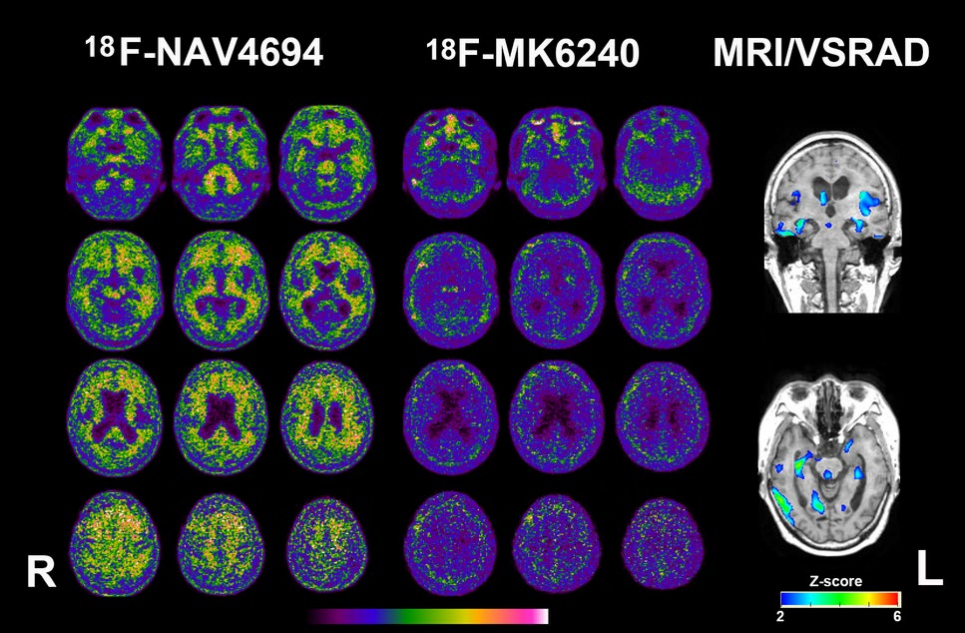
PET and MRI of a woman in her late 70s with Alzheimer’s and concomitant suspected non-Alzheimer’s pathologic change (A+T−N+). Amyloid PET Centiloid scale is 37. Tau PET is negative. The *Z*-score color map reveals significant atrophy in the mesial temporal regions with a *Z*-score of 2 or higher
